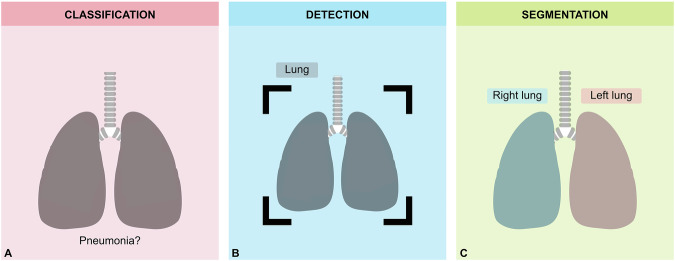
Three primary AI tasks in chest radiography analysis. A Classification: determining the presence of diseases such as pneumonia from chest X-ray images. B Detection: localising abnormalities within lung regions using bounding boxes or attention mechanisms. C Segmentation: precise pixel-level delineation of anatomical structures including left and right lung boundaries.
